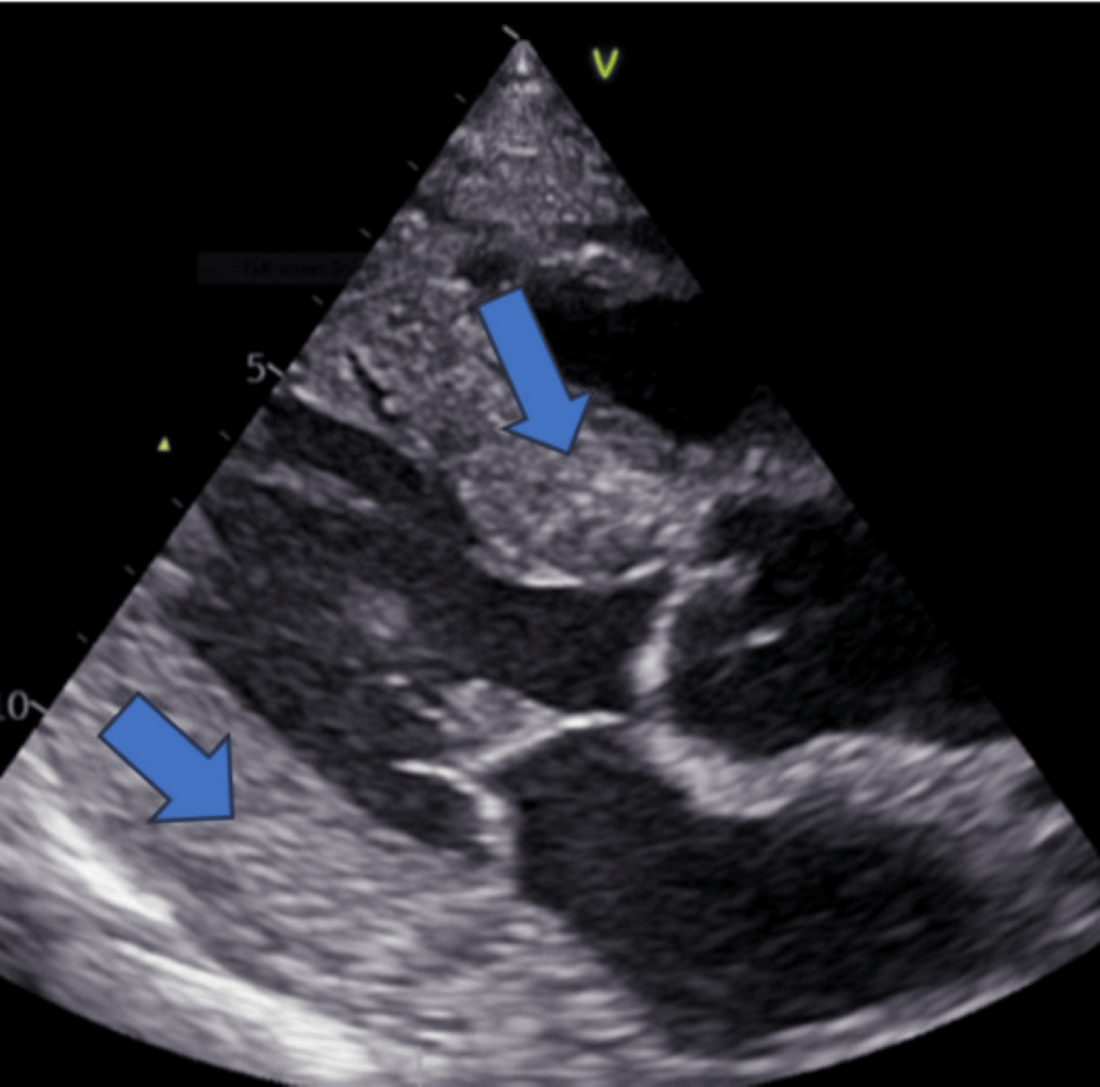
The echocardiogram showed biventricular hypertrophy with sparkling texture of myocardium (blue arrows).
